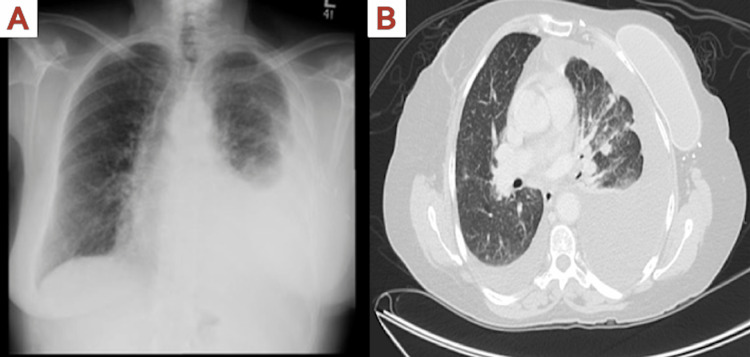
Imaging of pleural effusion (A) Chest X-ray showing pleural effusion. (B) CT in transverse plane showing pleural effusion
